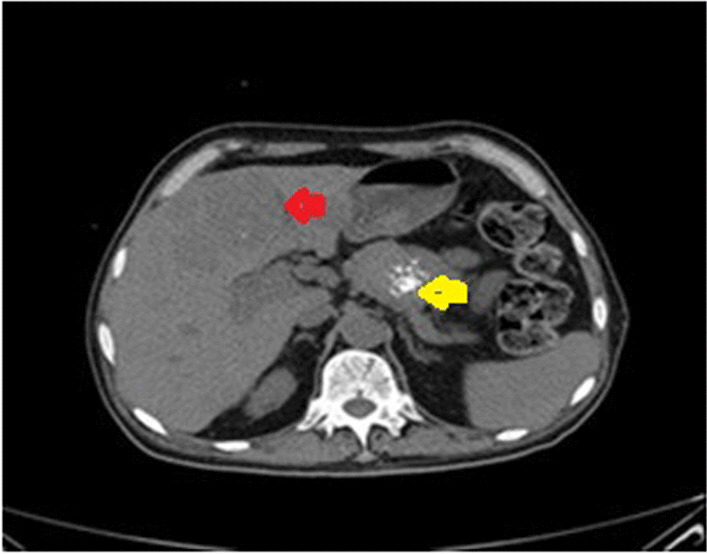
Abdominopelvic CT scan (axial view) without contrast showed a heterogeneous solid mass lesion, which measured 51 mm × 47 mm in the body of the pancreas and contained calcification (yellow arrow). The liver had heterogeneous parenchymal density with multiple varying size hypodense lesions in both lobes; the largest measurement was 73 mm × 67 mm in the left lobe (red arrow) and the foci of calcification in the mentioned lesion can be seen
